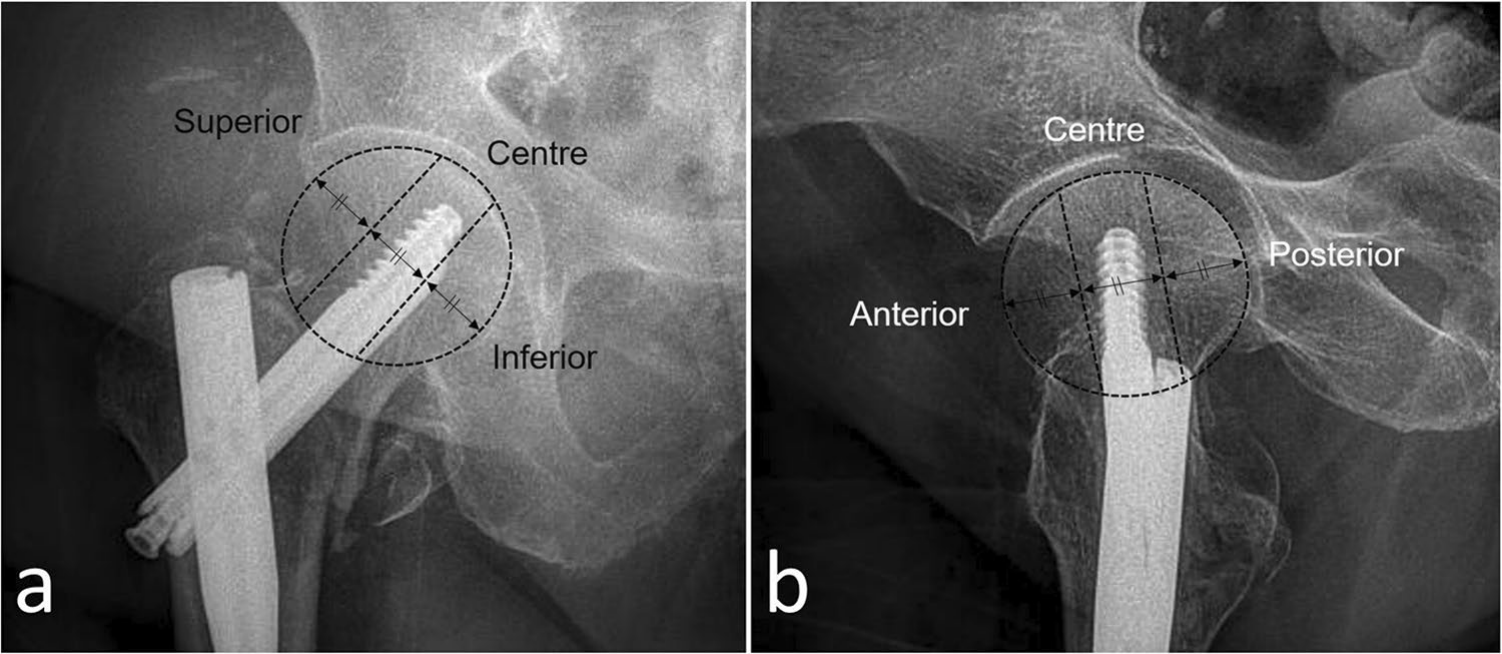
Determination of the lag screw quadrant. The femoral head was divided into three equal parts in AP and lateral planes in the direction of the neck axis. Superior, centre, and inferior quadrants on AP radiograph (a). Anterior, centre, and posterior quadrants on lateral radiograph (b)
